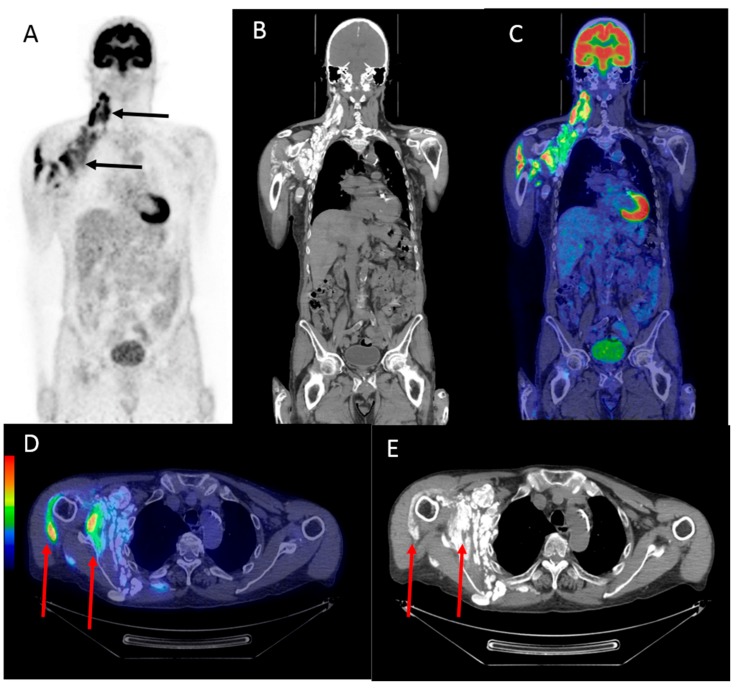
The maximum intensity projection (MIP) of the ^18^F-Fludeoxyglucose (^18^F-FDG) positron emission tomography (PET) image in the anterior view (A) showing multiple lesions with high ^18^F-FDG uptake located on the right side of the neck, around the right shoulder, and in the right axillar region (black arrows). A coronal computed tomography (CT) image (B) and a fused coronal image (C) of the head, neck, and upper body showing the same regions with high ^18^F-FDG uptake seen in the anterior view (A). Fused transversal images (D) and transversal CT images (E) showing the intramuscular high ^18^F-FDG uptake in the right axillary region (red arrows).
